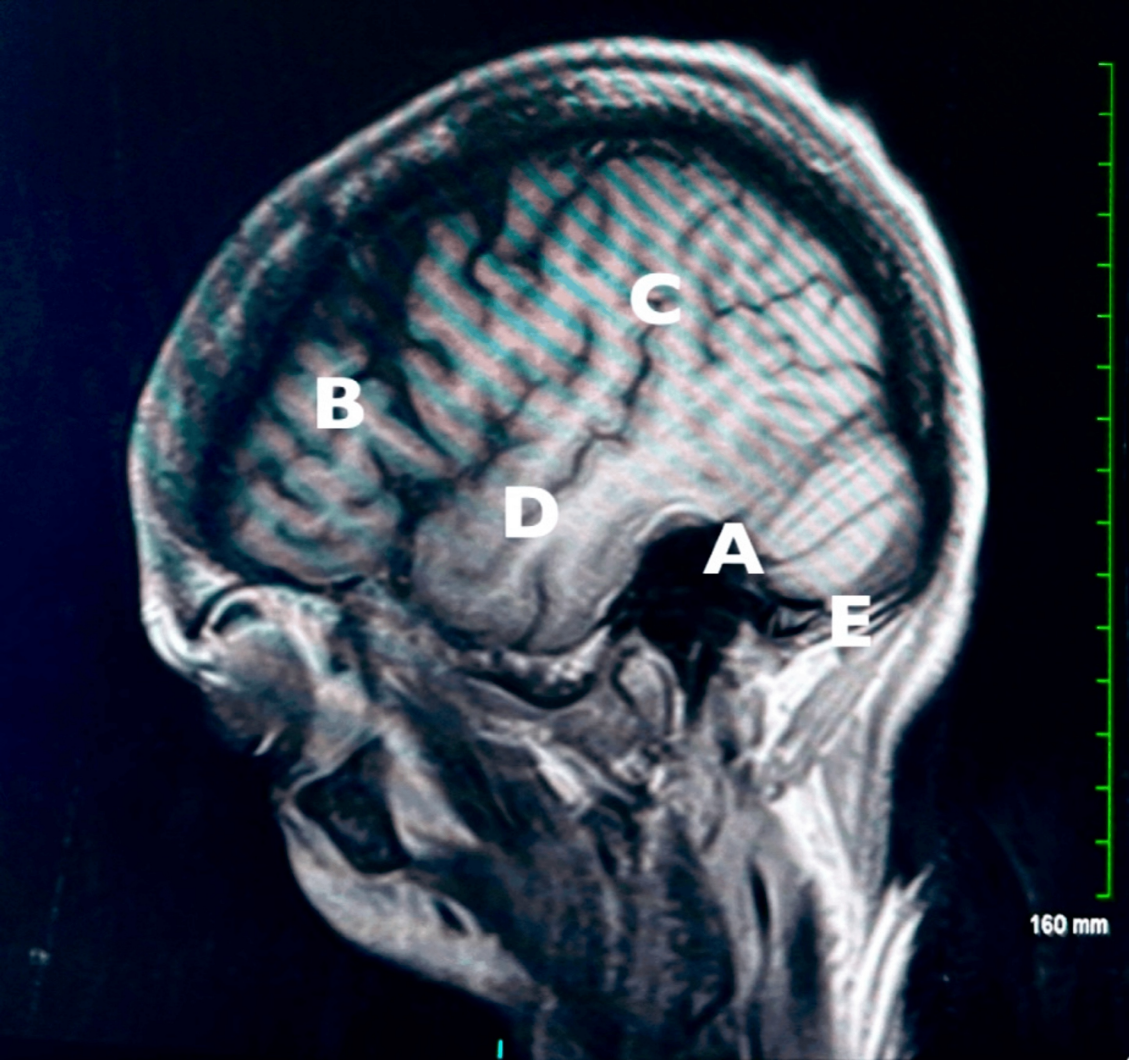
Sagittal MRI of the brain demonstrating suspected stage 4 metastasis of follicular lymphoma transformed to DLBCL in the left cerebellar hemisphere The lesion is seen in the occipital lobe (A). Labeled structures include the frontal lobe (B), parietal lobe (C), temporal lobe (D), and cerebellum (E). DLBCL: diffuse large B-cell lymphoma
